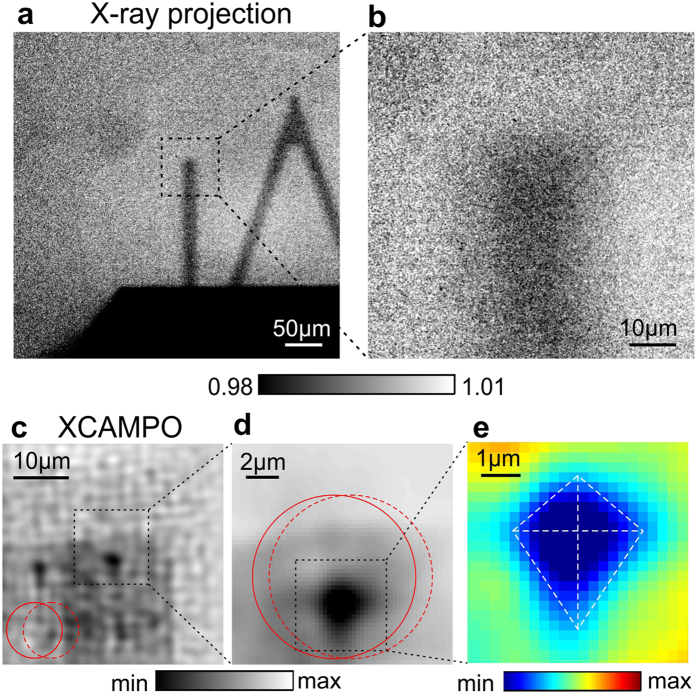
Grid-enhanced XCAMPO imaging of a weakly absorbing object (an AFM cantilever). (a) Standard X-ray projection image obtained with the optic but without the grid. (b) Zoomed-in view of (a). (c) A coarse grid-enhanced XCAMPO reconstruction performed to localize the AFM tip. (d) A fine grid-enhanced XCAMPO scan of the AFM tip. (e) Zoomed-in view of (d), shown using a false-colour scale for better visualization. The diamond-like shape represents a wire-frame image of the AFM tip. The centres of the red circles indicate two successive scanning positions, and their diameters reflect the focal spot size.
